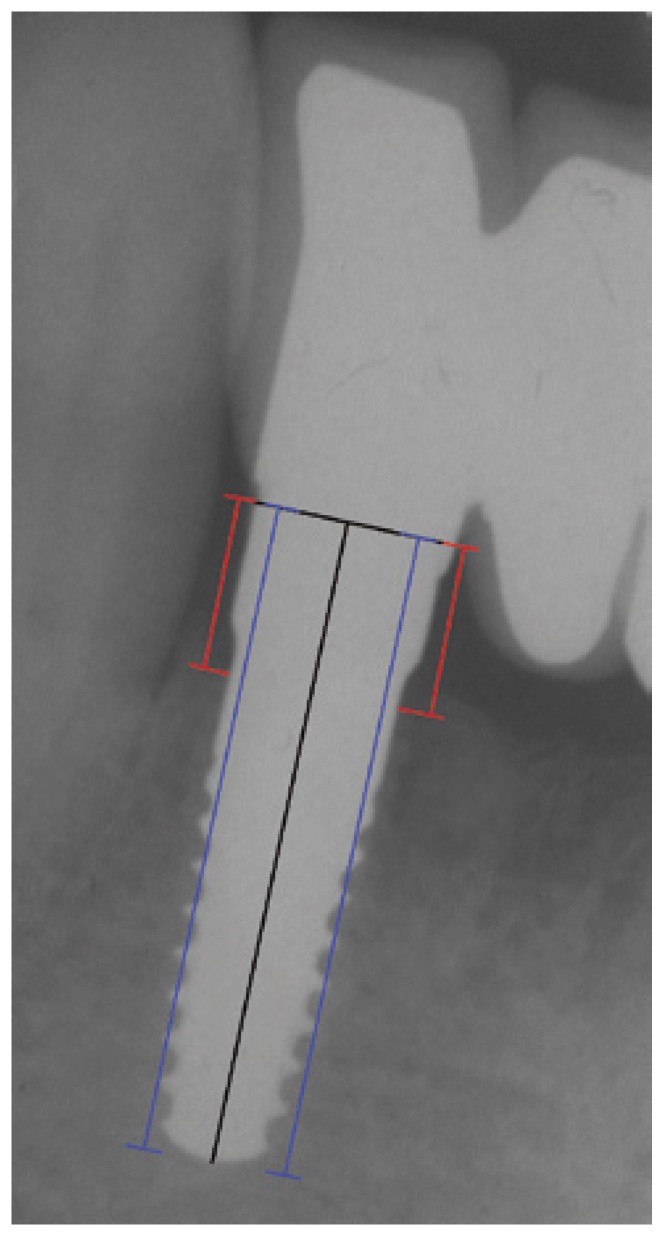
Twenty-four months after surgery: radiographic control of a zirconia dental implant positioned in 4.2. Assessment of the distance from the shoulder to the first bone-to-implant contact on digitalized radiographs (red lines). The blue lines refer to implant length.
